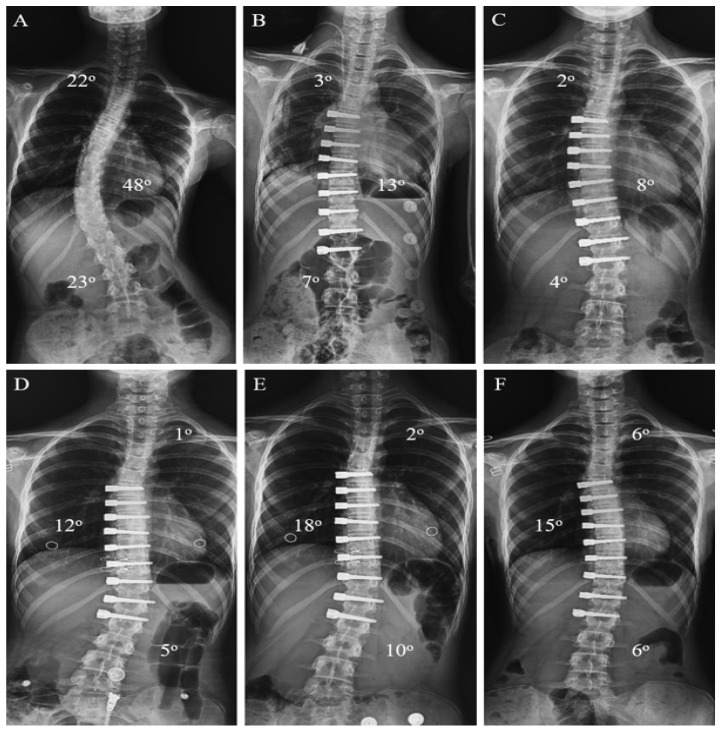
Anteroposterior radiograph of a patient who developed overcorrection complications. ((A): Preoperative radiography of a patient aged 11 years and 9 months; (B): First postoperative day radiography after AVBT surgery; (C): Postoperative sixth-month radiography; (D): One-year postoperative radiograph; (E): 1.5-year postoperative radiograph, patients aged 13 years and 3 months; (F): Eighth month of postoperative radiography after revision surgery, patient aged 14 years).
